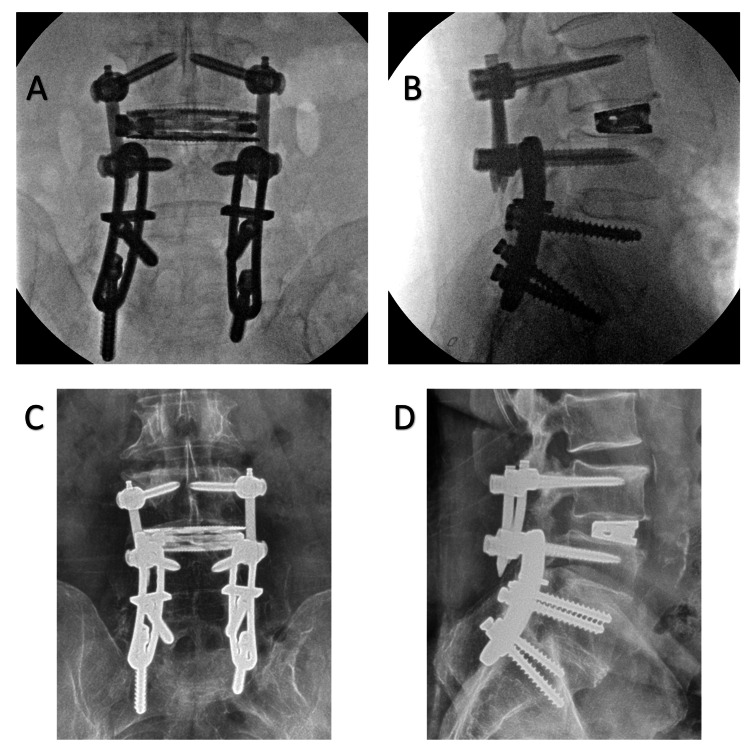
(A-B) Intra-operative AP (A) and lateral (B) fluoroscopic images demonstrating the final construct. (C-D) Upright AP (C) and lateral (D) radiographs at one-year follow-up reveal intact implants without suggestion of loosening or failure AP: anteroposterior
